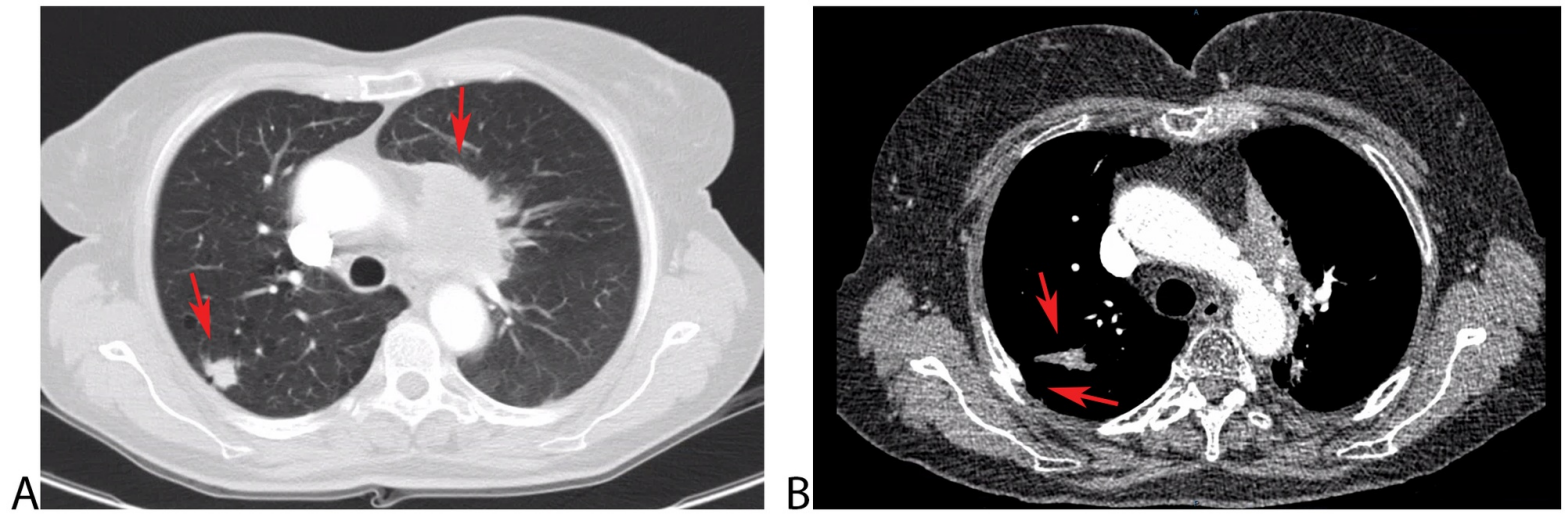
Computed tomography (CT) before and after first irradiation A) Transversal slice of both tumors on the CT scan 6.5 years ago at the time of diagnosis; large paramediastinal tumor on the left with some invasion in the aortopulmonary window (maximum size 65 mm) and second tumor on the dorsal side of the right upper lobe (maximum size 18 mm); B) CT of the thorax, transversal slice, two years after first irradiation. This scan shows a soft tissue component at the place of the right tumor and a fracture on the fifth right rib at the same level.
